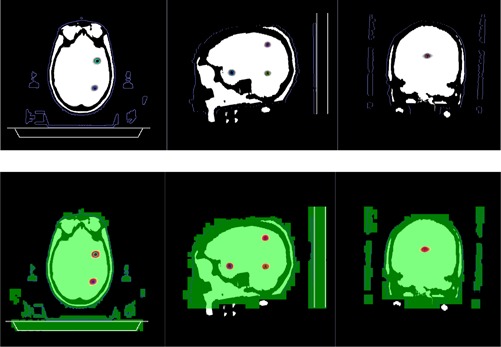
Co‐registration of postirradiation MRI images with planning CT and its associated RTstructures and RTdose calculated by ABMP. Color circles are the planning target volumes. The black areas on the MRI image are the actual irradiated volumes. The red color wash areas are the medium‐high dose regions (<5% relative dose) in the plan.
